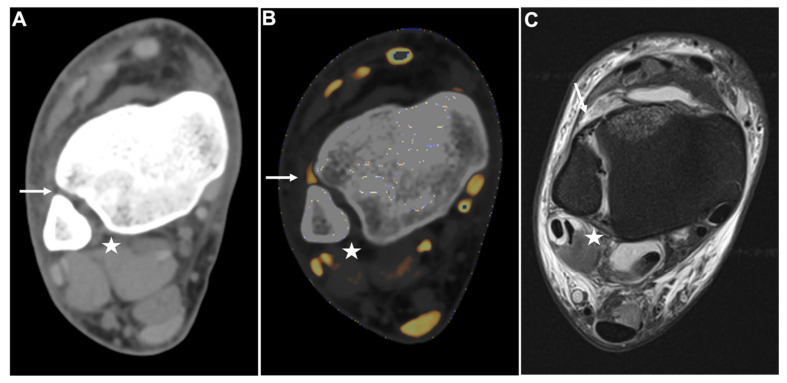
PTIFL strain. Standard axial unenhanced grayscale CT (A), color-coded collagen reconstructions (B), and proton density-weighted unenhanced MRI series with fat saturation (C) of a patient with an ankle sprain. While the ATIFL (arrow) and PTIFL (star) are visible in the grayscale images, no diagnostic assessment of both ligaments is accurately possible. After collagen mapping, the ATIFL is visible and intact (arrow). However, no collagen signal can be acquired for the PTIFL (star), suggestive of rupture. MRI images show an intact ATIFL (arrow) and a partial tear of the PTIFL (star), which likely caused a drop of the collagen content below acquirable thresholds. Abbreviations: ATIFL, anterior tibiofibular ligament. PTIFL, posterior tibiofibular ligament.
